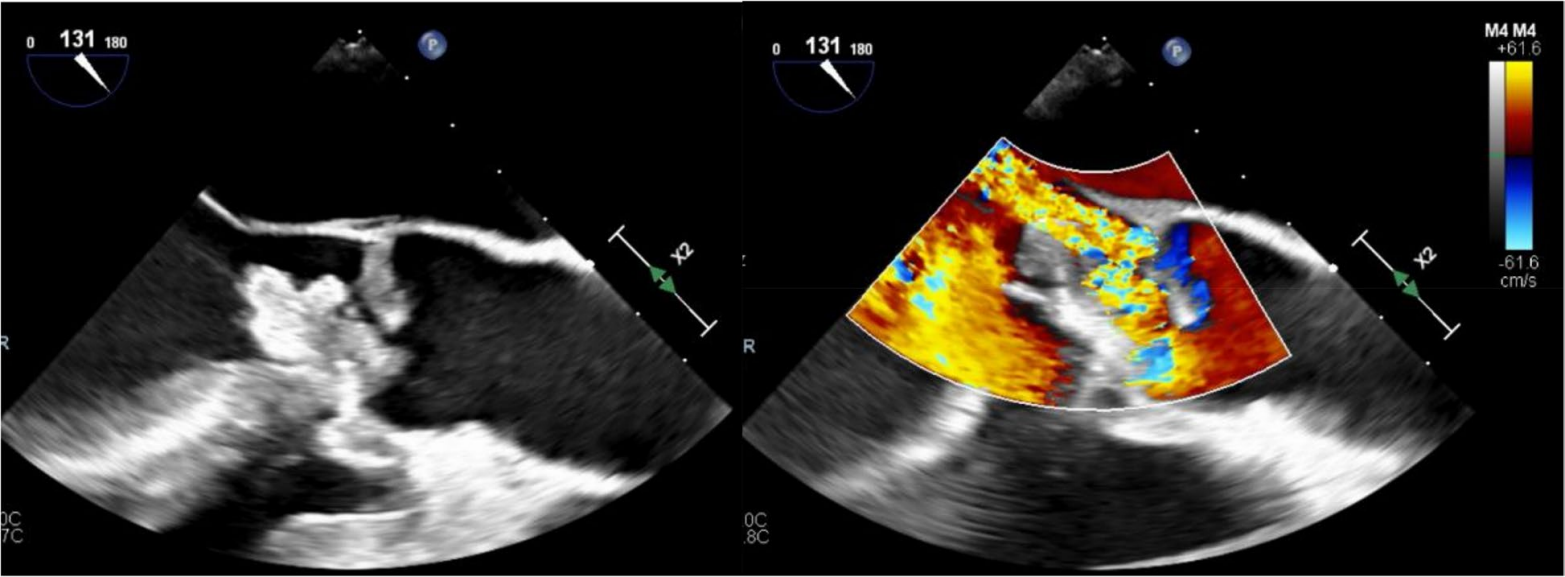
Transesophageal echocardiography findings. Still image on the left demonstrating a large mobile vegetation on his bicuspid aortic valve, while the image on the right is a still Doppler image showing significant aortic regurgitation. Images provided by Ethan Senser, MD
